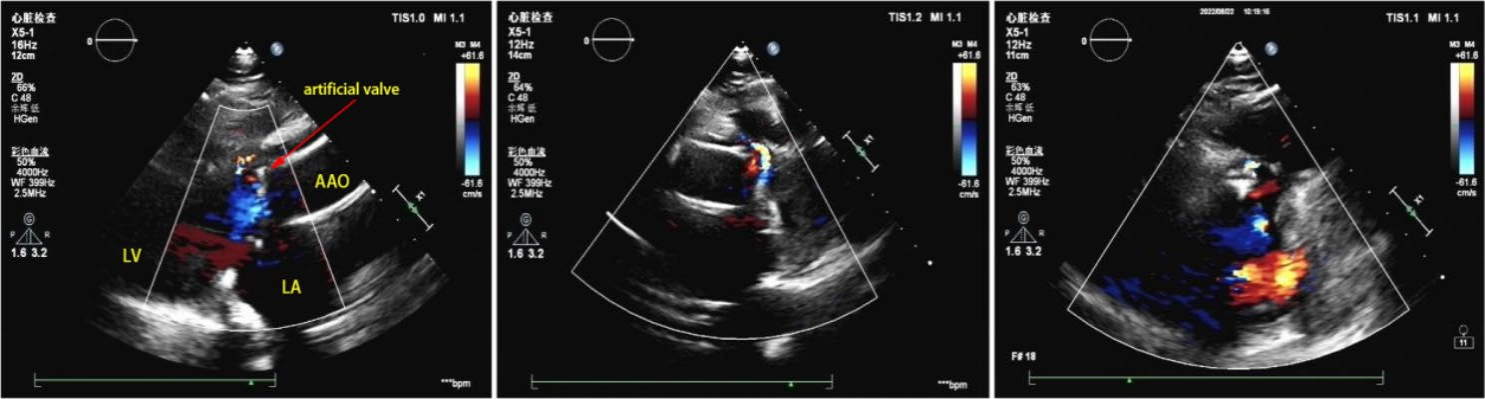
Post-operation echocardiography of the patient. A 4-mm gap between the artificial ascending aorta and the autologous vessel could be detected. The aortic valve was an artificial mechanical valve (yellow arrow) with a fixed mechanical annulus at approximately 12 o’clock, with a 3-mm spacing between the artificial and autologous annuli. The mitral valve was seen to have a strong echogenic forming ring. LV, left ventricle; LA, left atrium; AAO, ascending aorta
